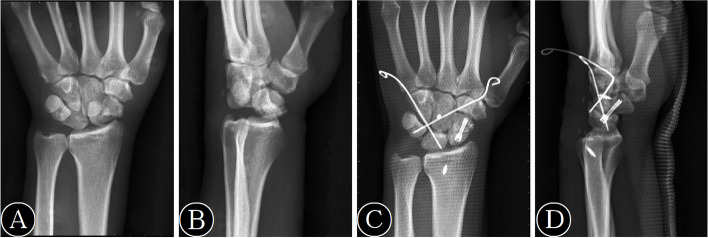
A 54-year-old man with right TSPFD. A Preoperative anteroposterior X-ray. B Preoperative lateral X-ray. C Postoperative anteroposterior X-ray. D Postoperative lateral X-ray
